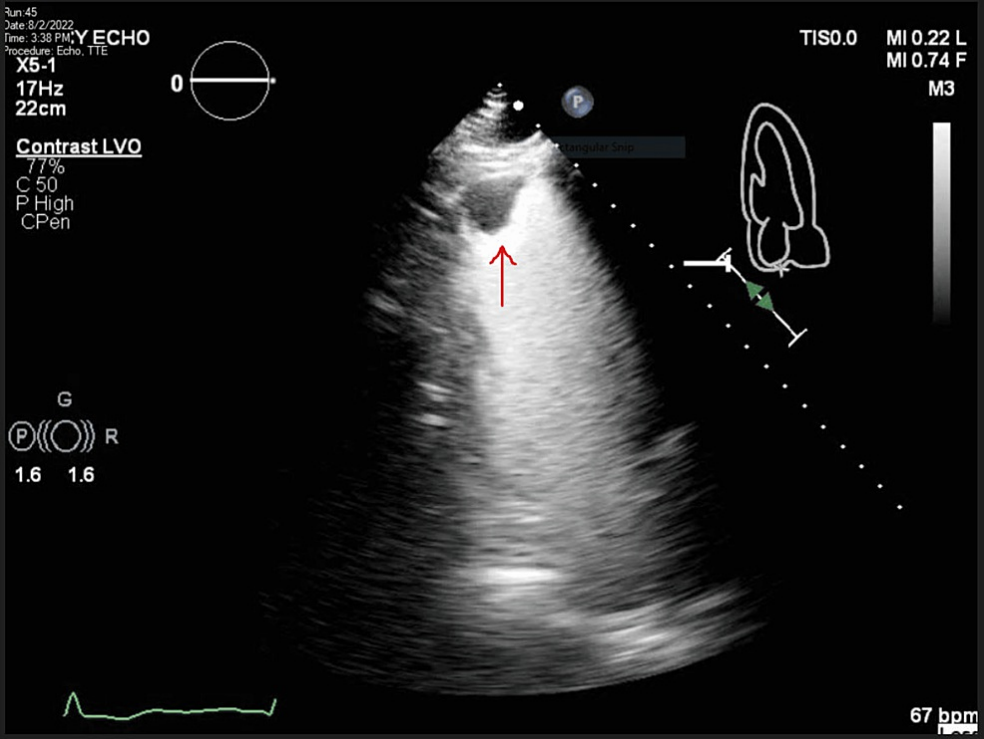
Apical two-chamber view on TTE with contrast enhancement demonstrating persistent LV apical mural thrombus TTE: Transthoracic echocardiogram; LV: left ventricle.
